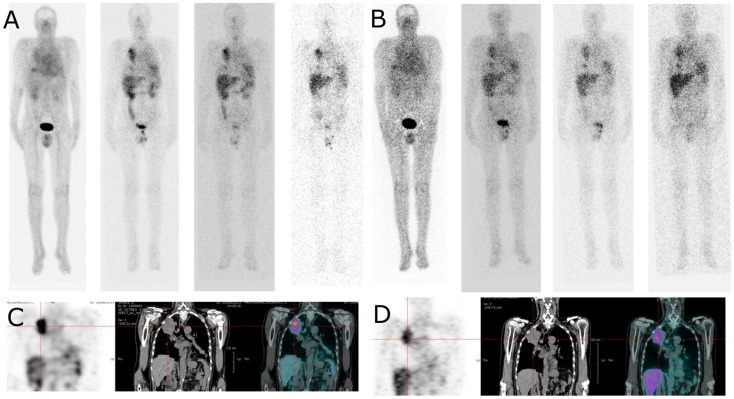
Whole body and SPECT/CT images of patient 4 (cohort II). Whole body images (anterior view) were acquired 4, 24, 48, and 72 h after injection of ^111^In-labelled IMP288 (A) and ^177^Lu-labelled IMP288 (B). Patient 4, included in the cohort II, had NSCL and a CEA level of 275 ng/mL. These images clearly shows lung tumor targeting in whole body and SPECT/CT images [(C) with ^111^In-labelled IMP288 and (D) with ^177^Lu-labelled IMP288]. According to RECIST criteria, the disease was considered as stable at 4 weeks, but progressive at 3 months. Patient 4 was the only patient with HAHA against TF2 > 50 ng/mL detected 1 month after the last TF2 injection.
